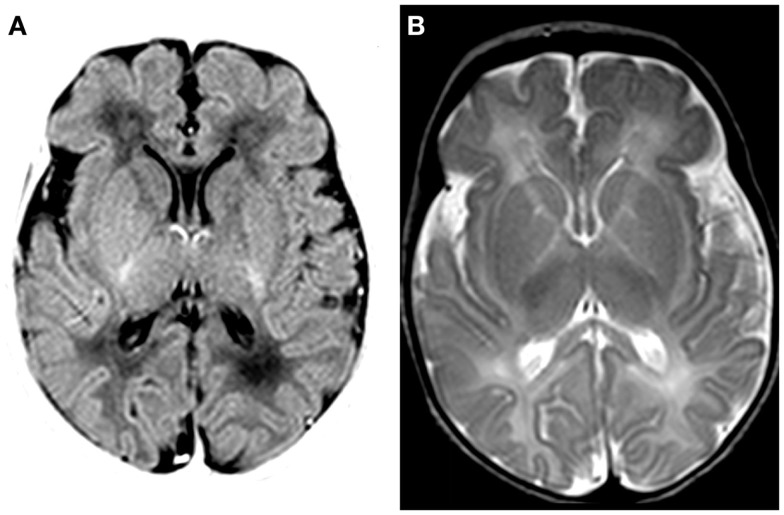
MRI findings on day 7. (A) T1-weighted MRI (inversion recovery) shows discrete swelling of the basal nuclei and thalamus. (B) T2-weighted MRI shows a decreased signal in basal nuclei and thalamus.
